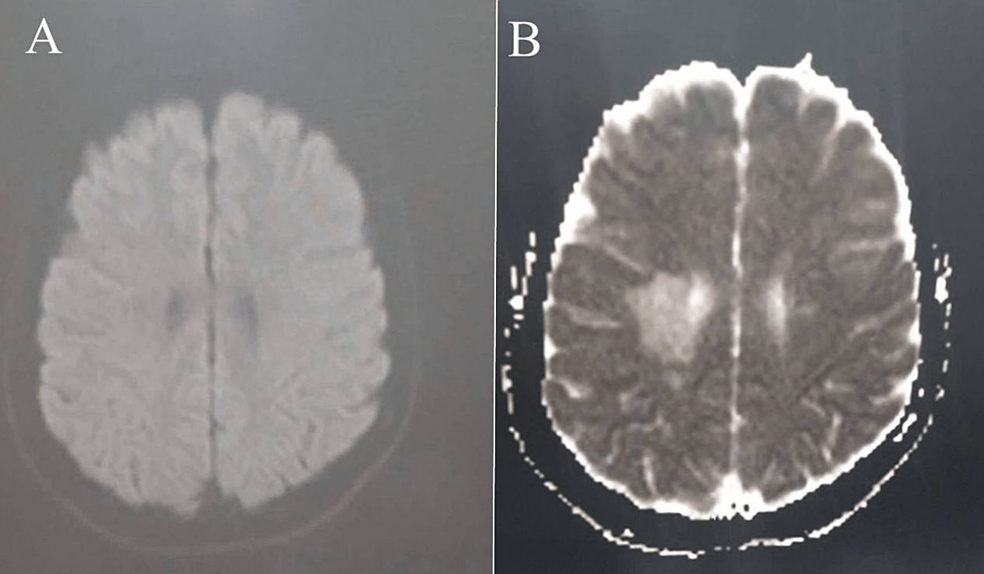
Brain Axial DWI/ADC MRI, show no restriction.
